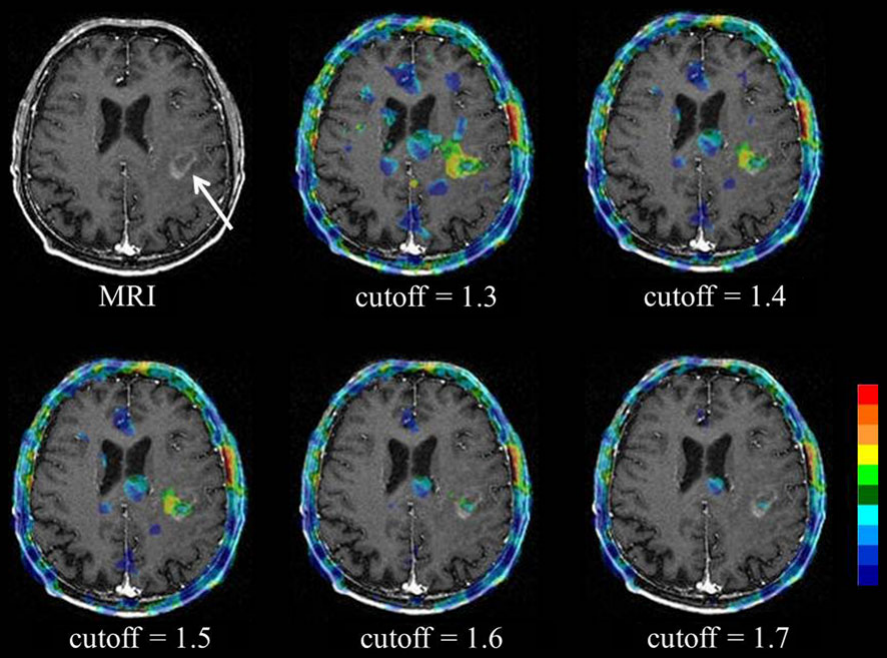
Cutoff value setting for retention index map. Low-grade (Grade II) astrocytoma on MR imaging (*arrow*). The background uptake and/or noise was widely remained for cutoff values of either 1.3 and 1.4. On the other hand, a part of the tumor uptake was removed for cutoff values of either 1.6 or 1.7
